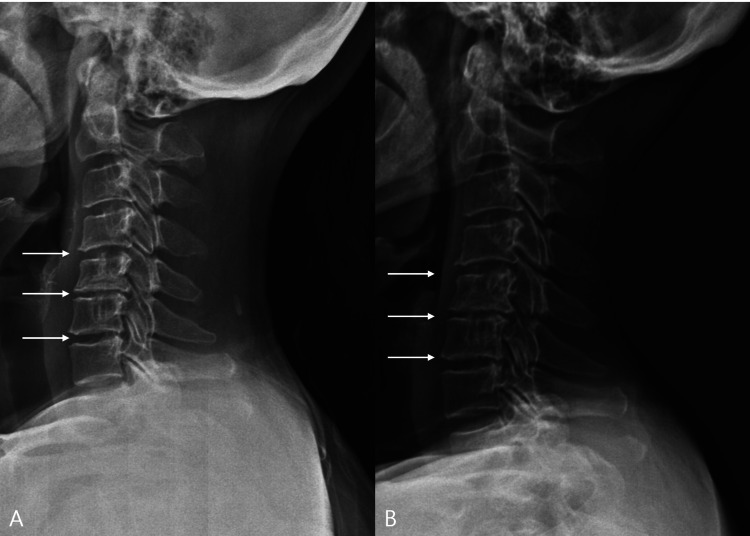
Lateral cervical spine radiographs at baseline and six-month follow-up. (A) Initial radiograph demonstrating multilevel disc space narrowing at C4-5, C5-6, and C6-7 (arrows). (B) Six-month follow-up radiograph of the same levels (arrows), showing relative preservation of disc spaces without clear radiographic progression and suggesting a possible subtle interval increase in disc height at the treated levels; however, this qualitative impression may have been influenced by positional variation.
